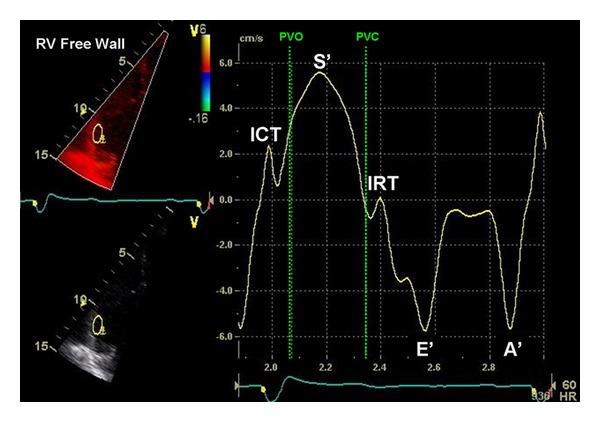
Color DTI waveforms obtained from the RV free wall describing the various phases in ventricular systole and diastole. There are 5 discrete waveforms occurring within the cardiac cycle, as seen on the Doppler display: (1) ICT velocity, (2) systolic (S0) velocity, (3) IRT velocity, (4) early diastolic velocity (E0), and (5) late diastolic velocity (A0). Dotted vertical lines represent PV opening (PVO) and PV closure (PVC). Copyright from Horton et al. (J Am Soc Echocardiogr 2009; 22: 776-792) with permission from the publisher, Copyright 2009, the Journal of the American Society of Echocardiography.
